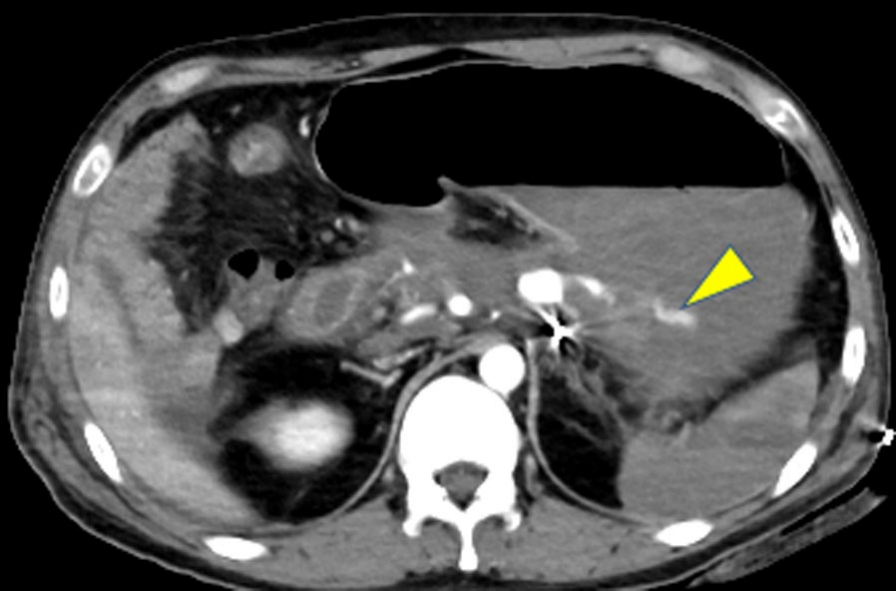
A second dynamic CT shows extravasation from the SAP into the stomach (triangle)
